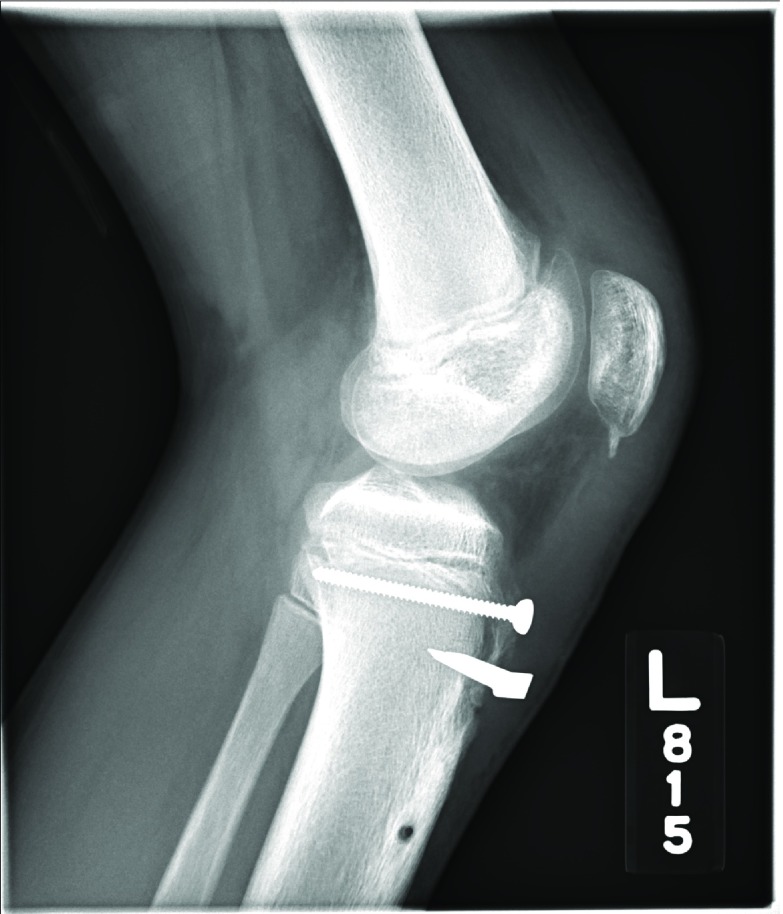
Postoperative radiographs of the patient described in Case 2. Surgical repair including screw and staple fixation of the tibial tubercle avulsion fracture is evident.
